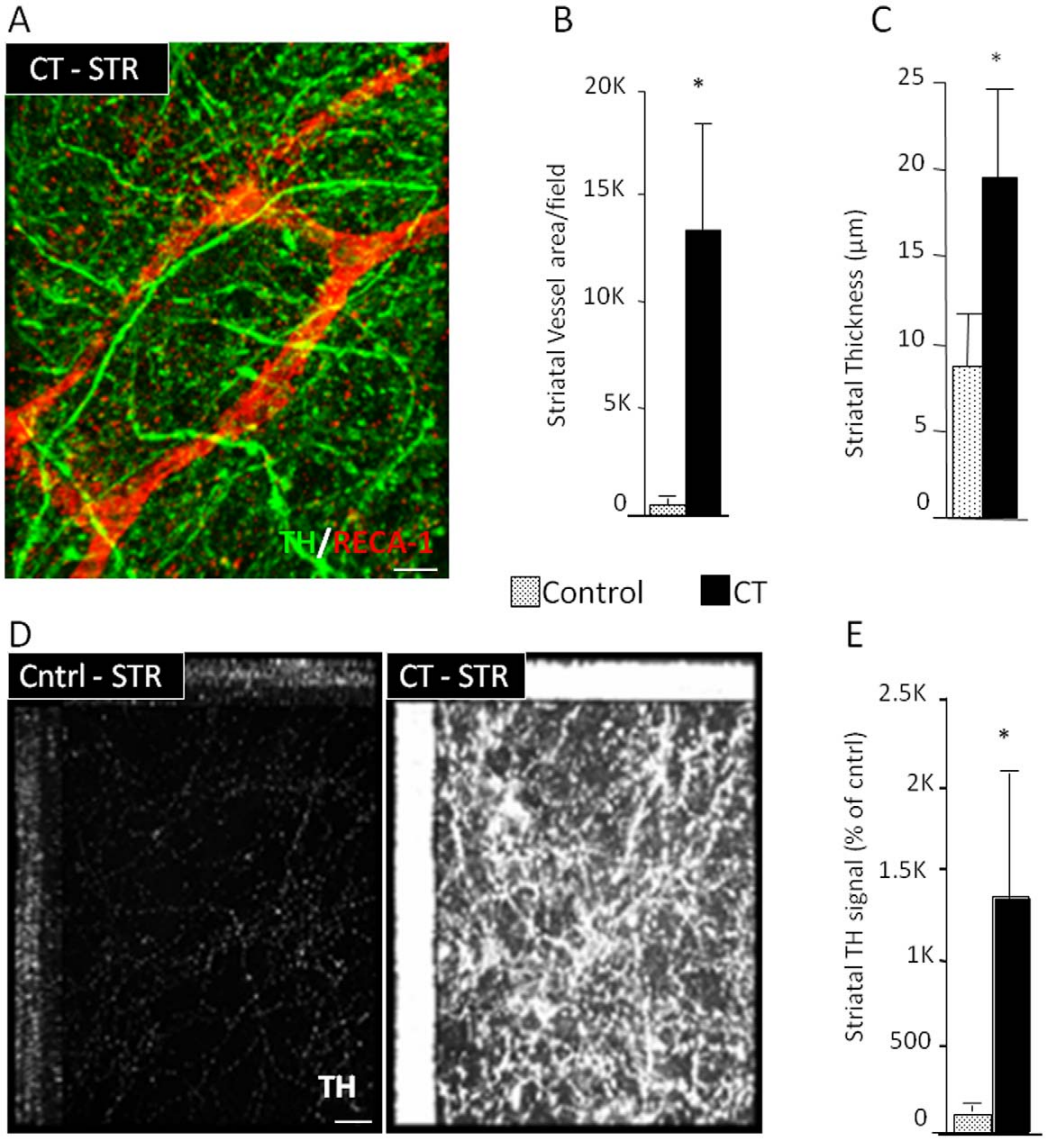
Increased vascular coverage and neuronal projections by angiogenic factors. (A, B) CT treatment of organotypic slice cultures (every 4 days for 2 weeks) retains the vasculature (confocal projection for the pan-endothelial marker RECA-1 and TH), (C) increases the thickness of the striatal portion of the slice, (D,E) promotes the sprouting of TH^+^ fibers from the S. Nigra section to the striatal section (2-weeks after control (BSA) and CT treatment). [Size bars: 20 µm].
